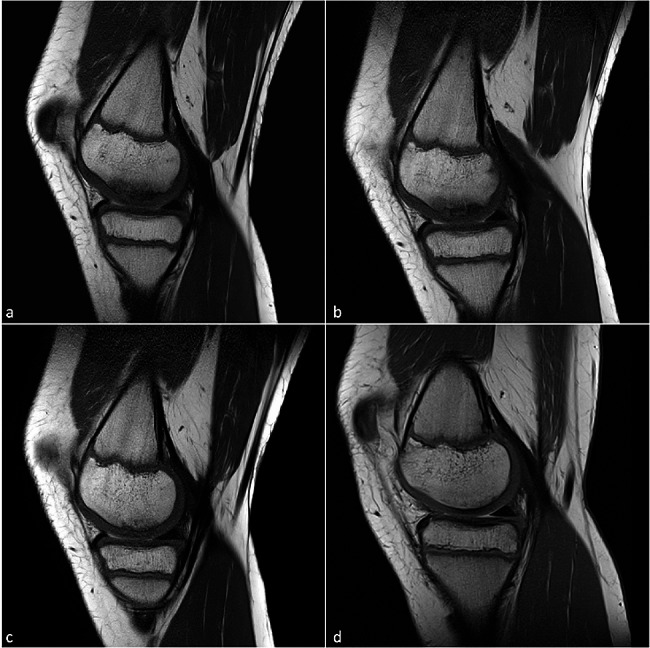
T1-weighted turbo spin echo sequences (T1-TSE) follow-up MR images of osteochondrosis dissecans of the right knee joint of twin 1 in the sagittal plane: from the stage at the onset of symptoms *a)*, there is a decrease in lesion size and perifocal edema after *b)* six months and *c)* fourteen months until complete consolidation of the lesion under conservative treatment after *d)* two years of follow-up. There is a decrease in reactive cartilage thickening with a continuous cartilage surface
